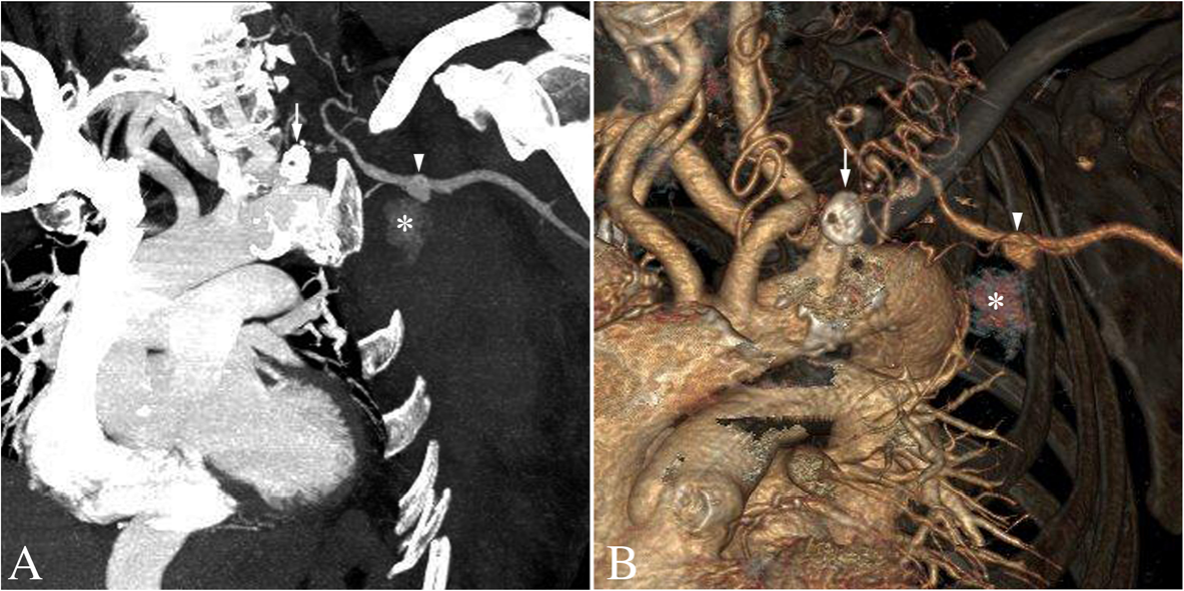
Contrast-enhanced computed tomography of aneurysm of the left subscapular artery. Oblique maximum intensity (A) and volume rendering (B) projections of contrast-enhanced computed tomography show a 1cm-diameter aneurysm of the left subscapular artery (arrowheads), associated with a blush of contrast agent (asterisks). More proximally, there is an extensively calcified chronic total occlusion of the proximal left subclavian artery (arrows). Of note, there is an aberrant origin of the right subclavian artery.
